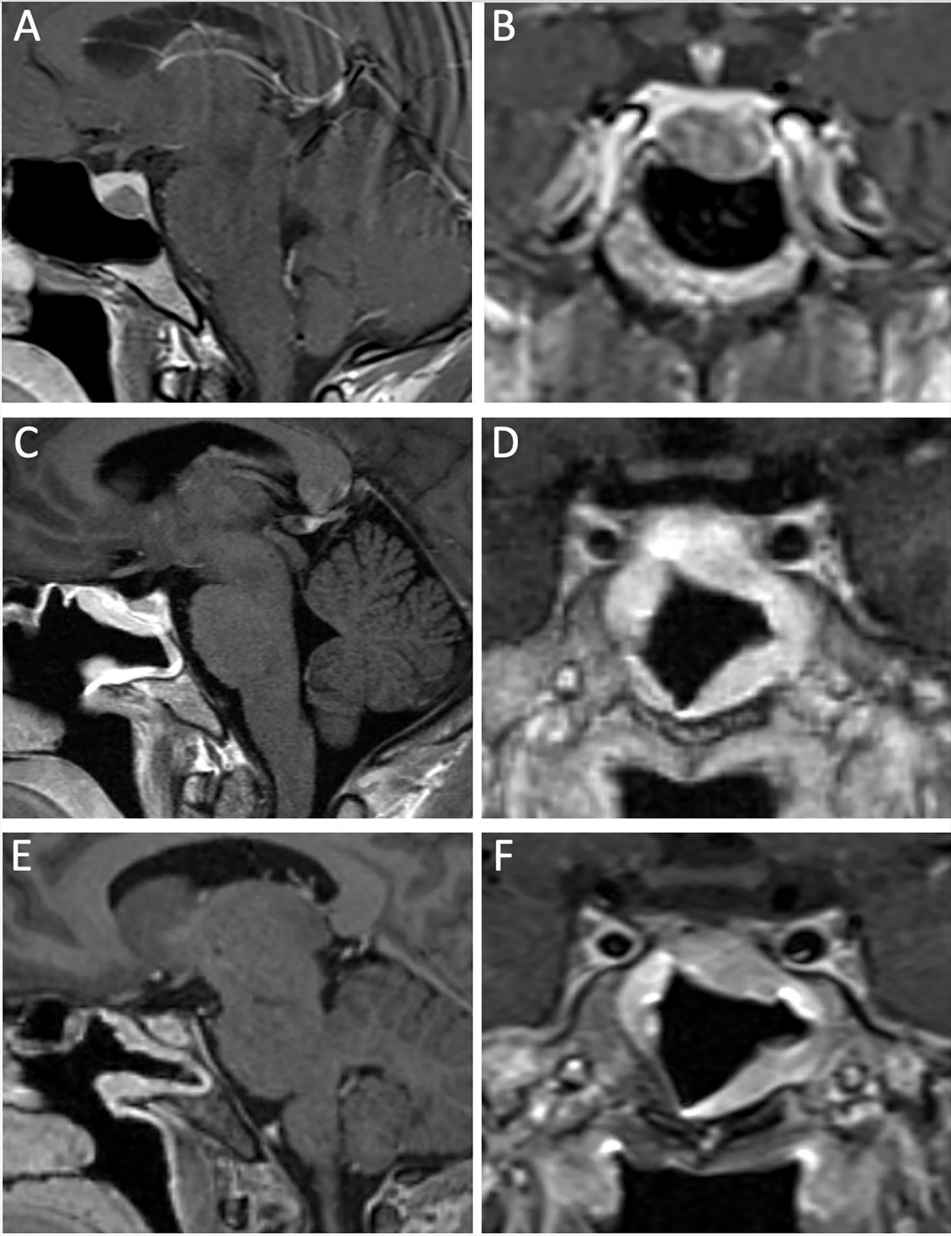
A 33-year-old man with a GH-secreting microadenoma. Preoperative MRI, T1-post gadolinium contrast, featuring sagittal (A) and coronal (B) views that demonstrate a small adenoma, confined to the pituitary sella. 3-month postoperative MRI, T1-post gadolinium contrast, sagittal (C) and coronal (D) views show gross total resection of the lesion, with well-vascularized nasoseptal flap. More than 1 year from surgery, there is no residual tumor as demonstrated by the sagittal (E) and coronal (F) MRI T1-post gadolinium contrast. The patient achieved biochemical cure after resection of the GH-secreting adenoma.
